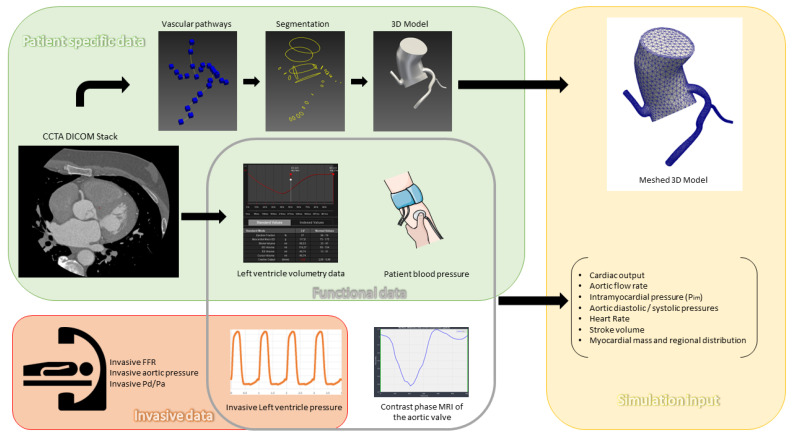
Patient data preprocessing: CCTA: Coronary Computed Tomography Angiography; DICOM: Digital; Imaging and Communications in Medicine; FFR: Fractional Flow Reserve; Pa: Aortic Pressure; Pd: Distal Coronary Pressure; MRI: Magnetic Resonance Imaging.
